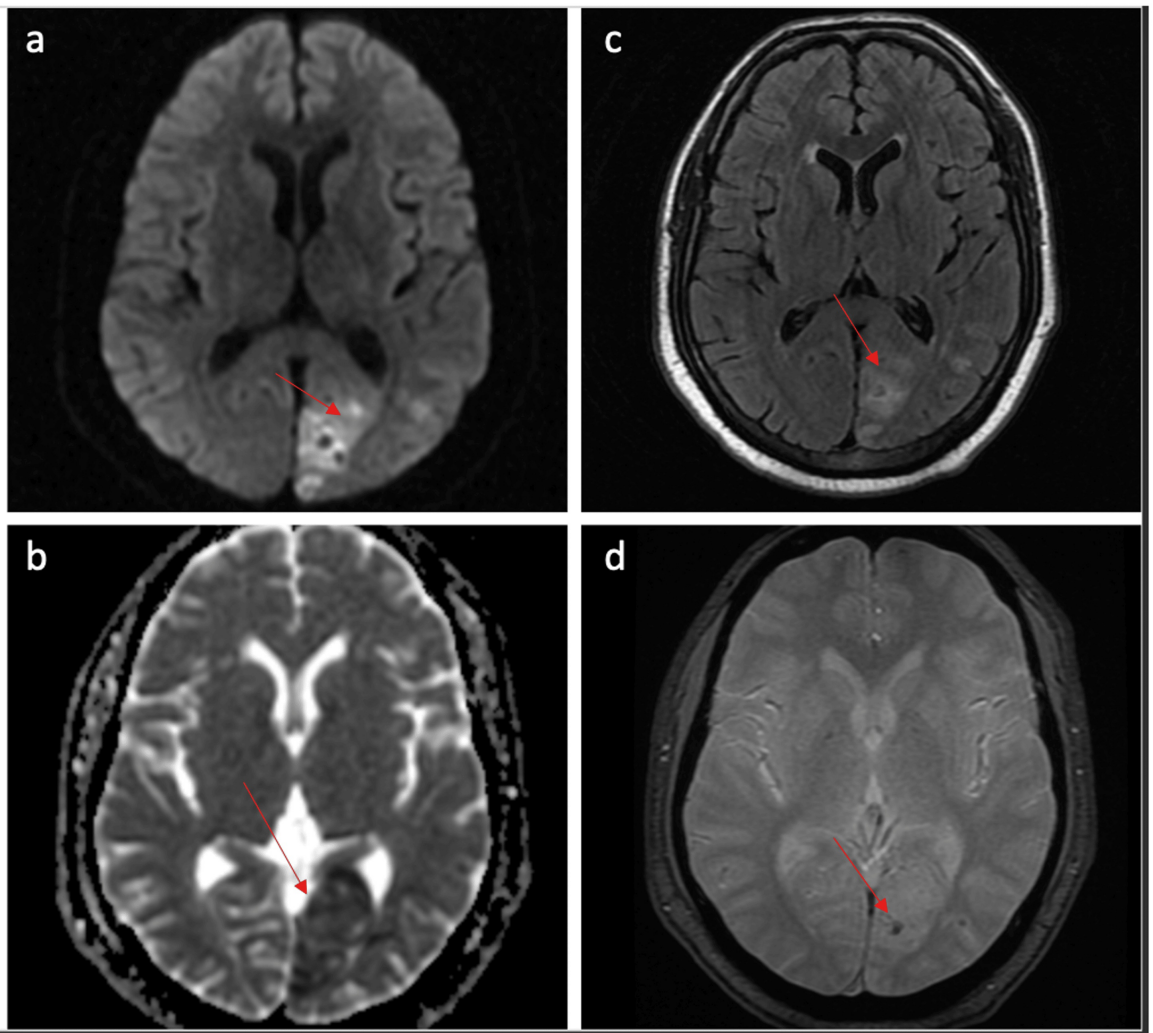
MRI brain a. Diffusion-weighted images(DWI) show an area of restricted diffusion in the left occipital lobe; b. Corresponding low value on apparent diffusion coefficient (ADC) mapping; c. The area is hyperintense on fluid-attenuated inverse recovery (FLAIR); d. Area of blooming on gradient echo (GRE) sequence consistent with microhemorrhage
